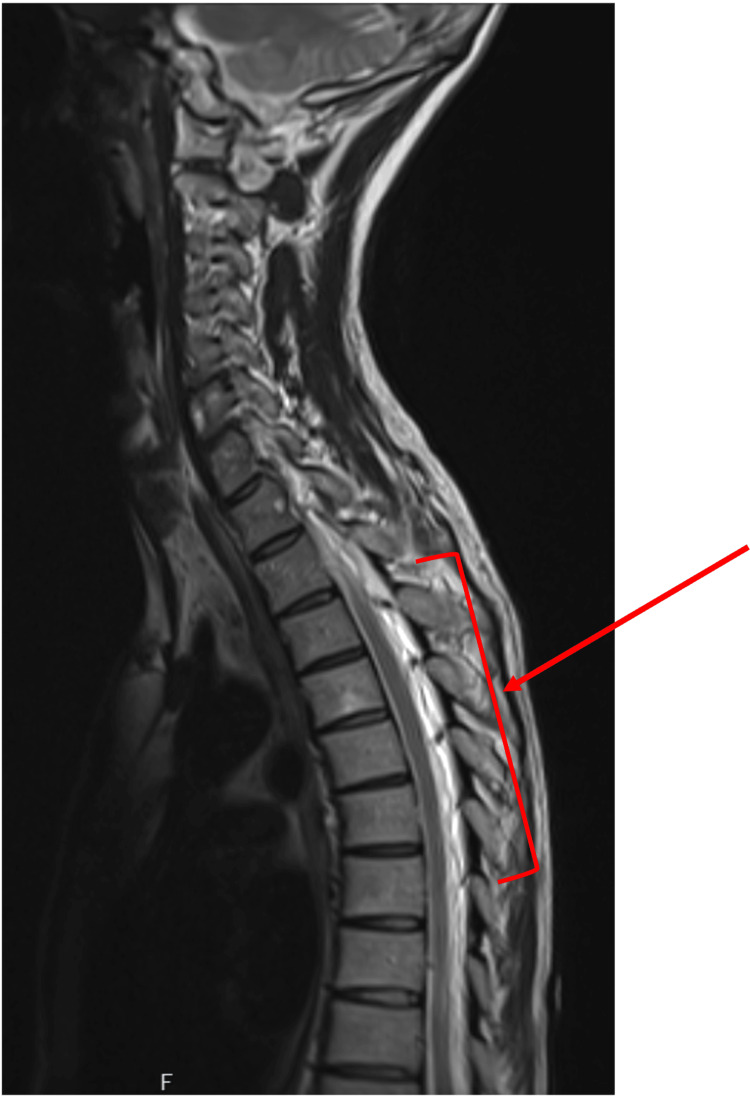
MRI of the whole spine showing congestion and tortuosity of the posterior epidural veins extending from the T2/T3 level down to the T8/T9 level
